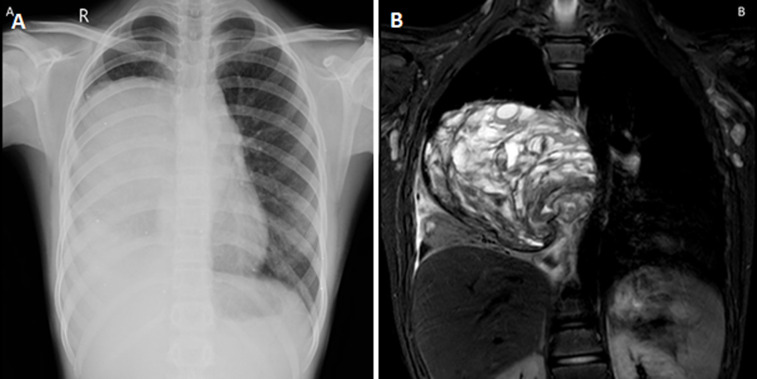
A) chest X-ray of the patient showing homogenous opacity in the right lung; B) MRI of the thorax showing solid cystic lesion in the right hemithorax with mild pleural effusion
